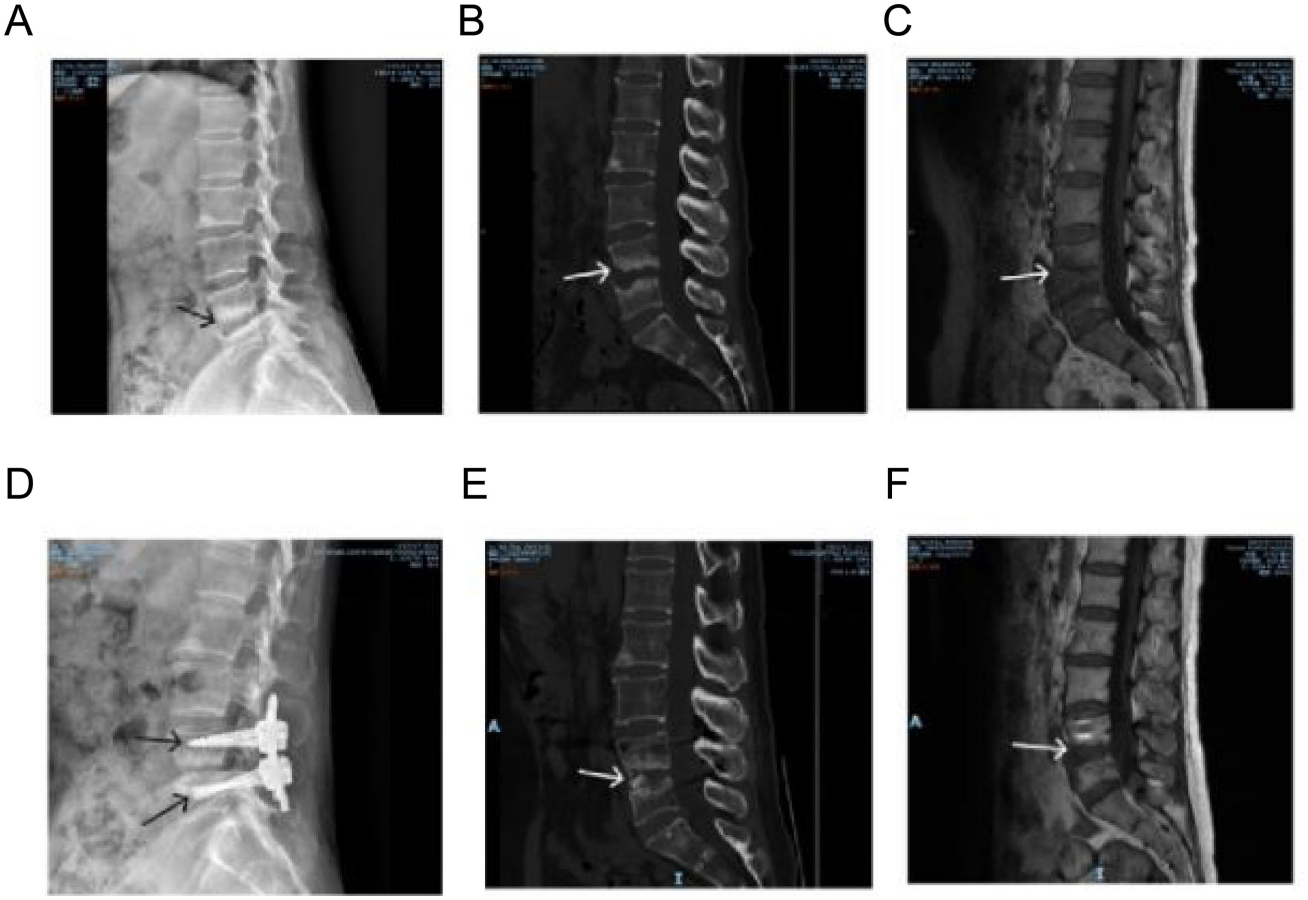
Imaging studies. (A) Preoperative X-ray image showing irregularities at the relative edges of L4-5 vertebrae, increased bone density, and mild posterior displacement of the L4 vertebra. (B) CT imaging reveals bone loss at the relative edges of the L4-5 vertebrae and increased density. (C) MRI image suggesting the presence of local bone loss. (D–F) Postoperative imaging at 18 weeks (X-ray, CT, MRI) shows no abnormal signals at the lesion site, indicating no recurrence of infection.
